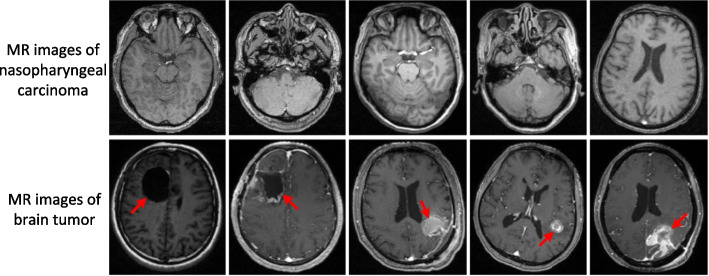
MR images of nasopharyngeal carcinoma and brain tumor. The first row is the images with nasopharyngeal carcinoma. The second row is the images with brain tumor. Usually there are some postoperative regions on brain tumor images that exhibit significant appearance variations and intensity variations
